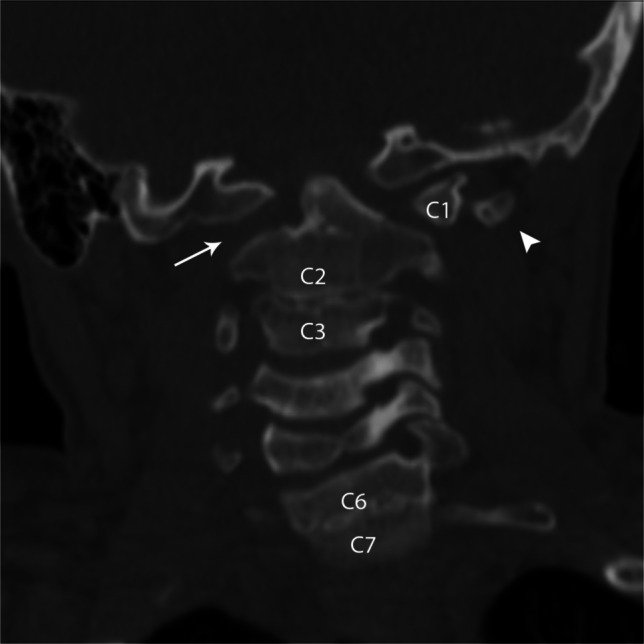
Coronal CT of the cervical spine in an 11-year-old female. Aplastic right C1 (*arrow*) with normal left C1 (*C1*) with normal left C1 transverse process (*arrowhead*). When C1 is aplastic, the transverse process is absent. Multiple segmentation anomalies of the cervical spine including C2 and C3 as well as C6 and C7
